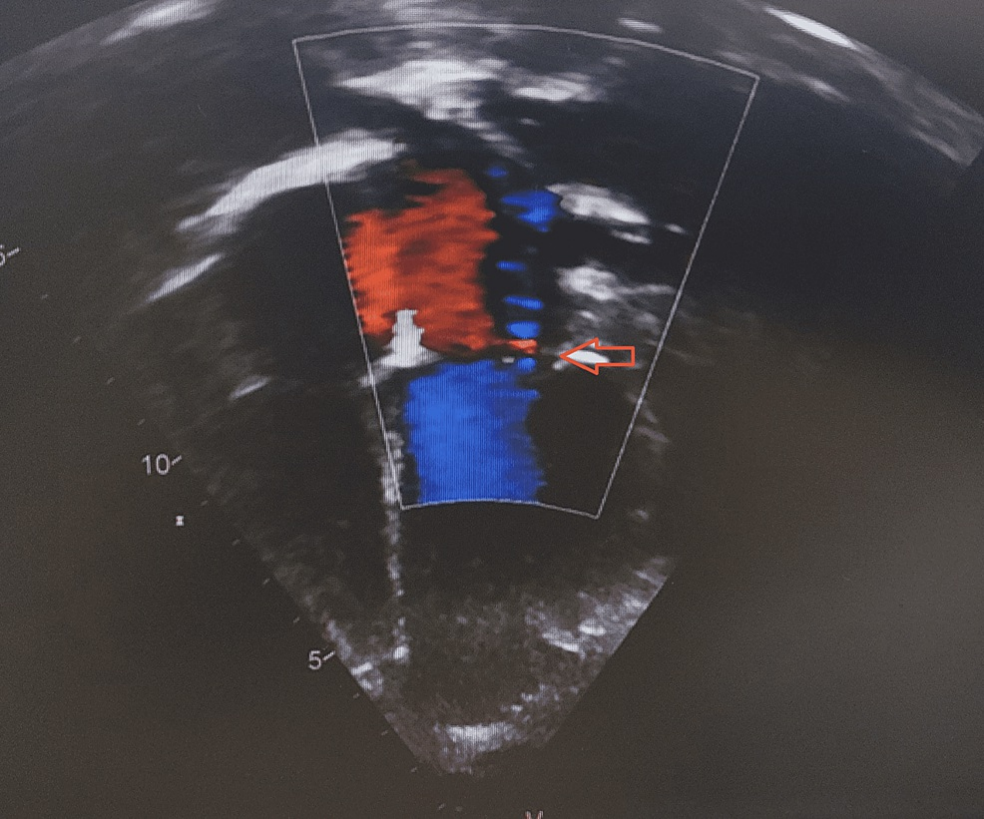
Echocardiography (Apical four-chamber view showing mild Mitral regurgitation)
